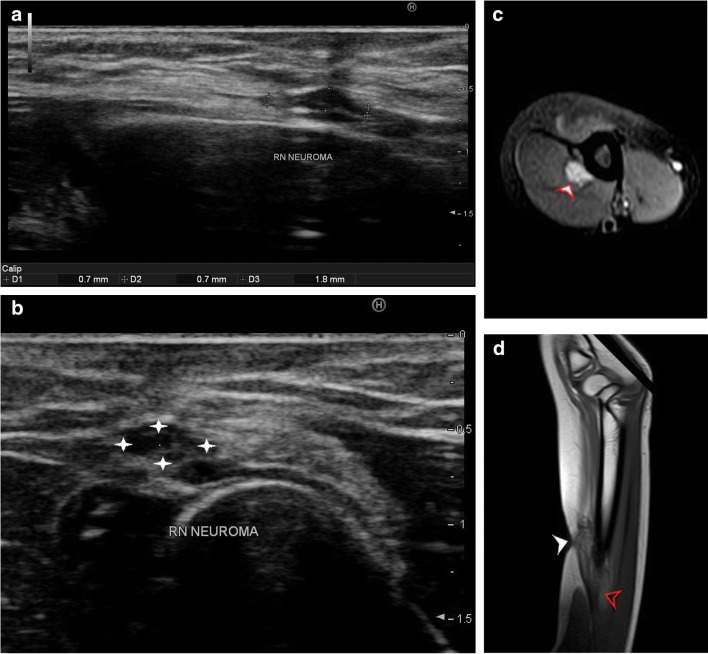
a Focal fusiform thickening of radial nerve is noted (D3 marker) with normal calibre proximally and distally (D1 and D2 markers). The nerve is hypoechoic at the site of enlargement of the nerve. This anatomical abnormality was noted just deep to the scar at the site of bite on the skin. b Short axis view of focal thickening suggestive of radial nerve neuroma (between cross marks) seen distal to the spiral groove. The nerve is seen lying between the brachialis and brachioradialis. c Axial PD fat saturated sequence of the same patient showing a well-defined ovoid hyperintense lesion (black arrowhead) in continuity with the radial nerve. As the lesion was noted distal to the spiral groove, after supply to the triceps, there is no signal intensity change in the triceps muscle signifying normal innervation to the muscle. d Sagittal PD sequence showing a fusiform hyperintense lesion (black arrowhead) corresponding with the ultrasound findings. The scar site on the skin can also be noted (white arrowhead)
